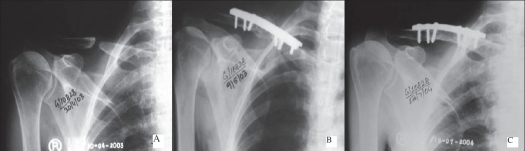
X-ray right shoulder anterior posterior view of a 51 years old female with 7 years old fracture clavicle. Showing. A) non-union of clavicle. B) fixation with DCP alongwith strut graft. C) 14 months old post operative X-rays
